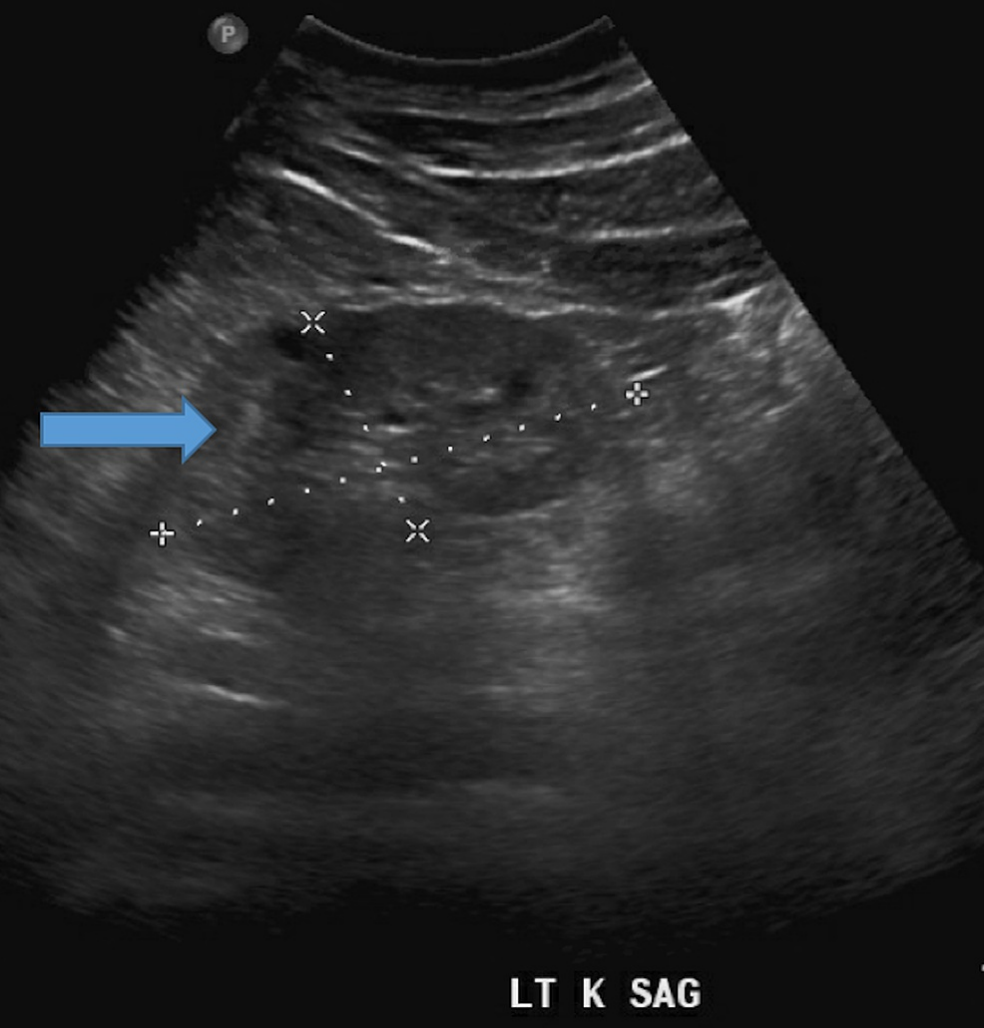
Ultrasound of the left kidney (blue arrow) measuring 8.67 by 4.09 cm. Left kidney (LT K), Saggital (SAG)
